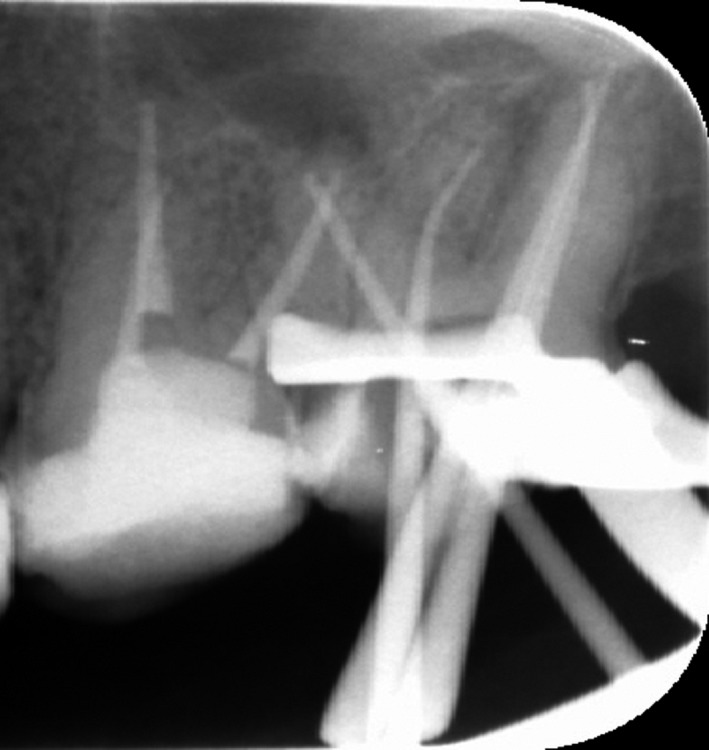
Master Apical Cone Radiograph
